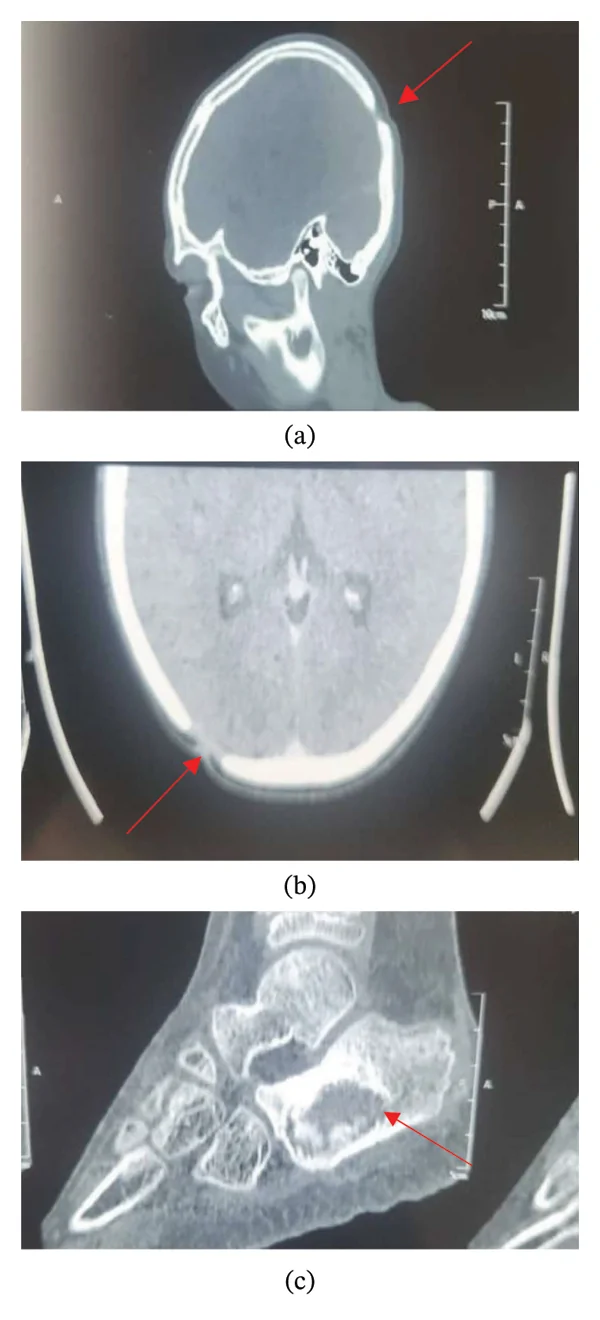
(a) Right parietal osteolytic lesion measuring 27 × 8.0 mm. Disappearance of the epidural thickening adjacent to the known lesion. (b) Right parietal osteolytic lesion measuring 27 × 8.0 mm. Disappearance of the epidural thickening adjacent to the known lesion. (c) CT of the left foot showing osteolytic lesion of the left calcaneus with cortical fracture (sagittal plane).
